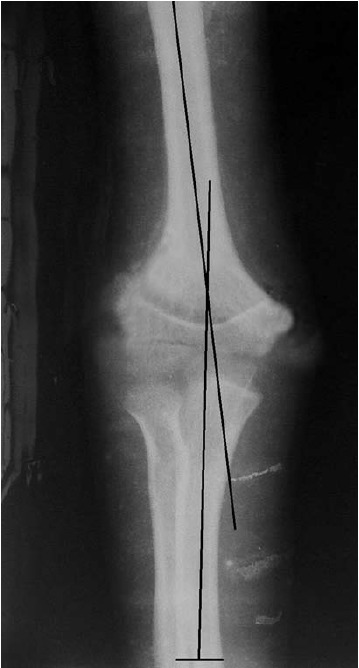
Fig. 2b: Lateral view radiograph at final follow up showing carrying angle F2b:
